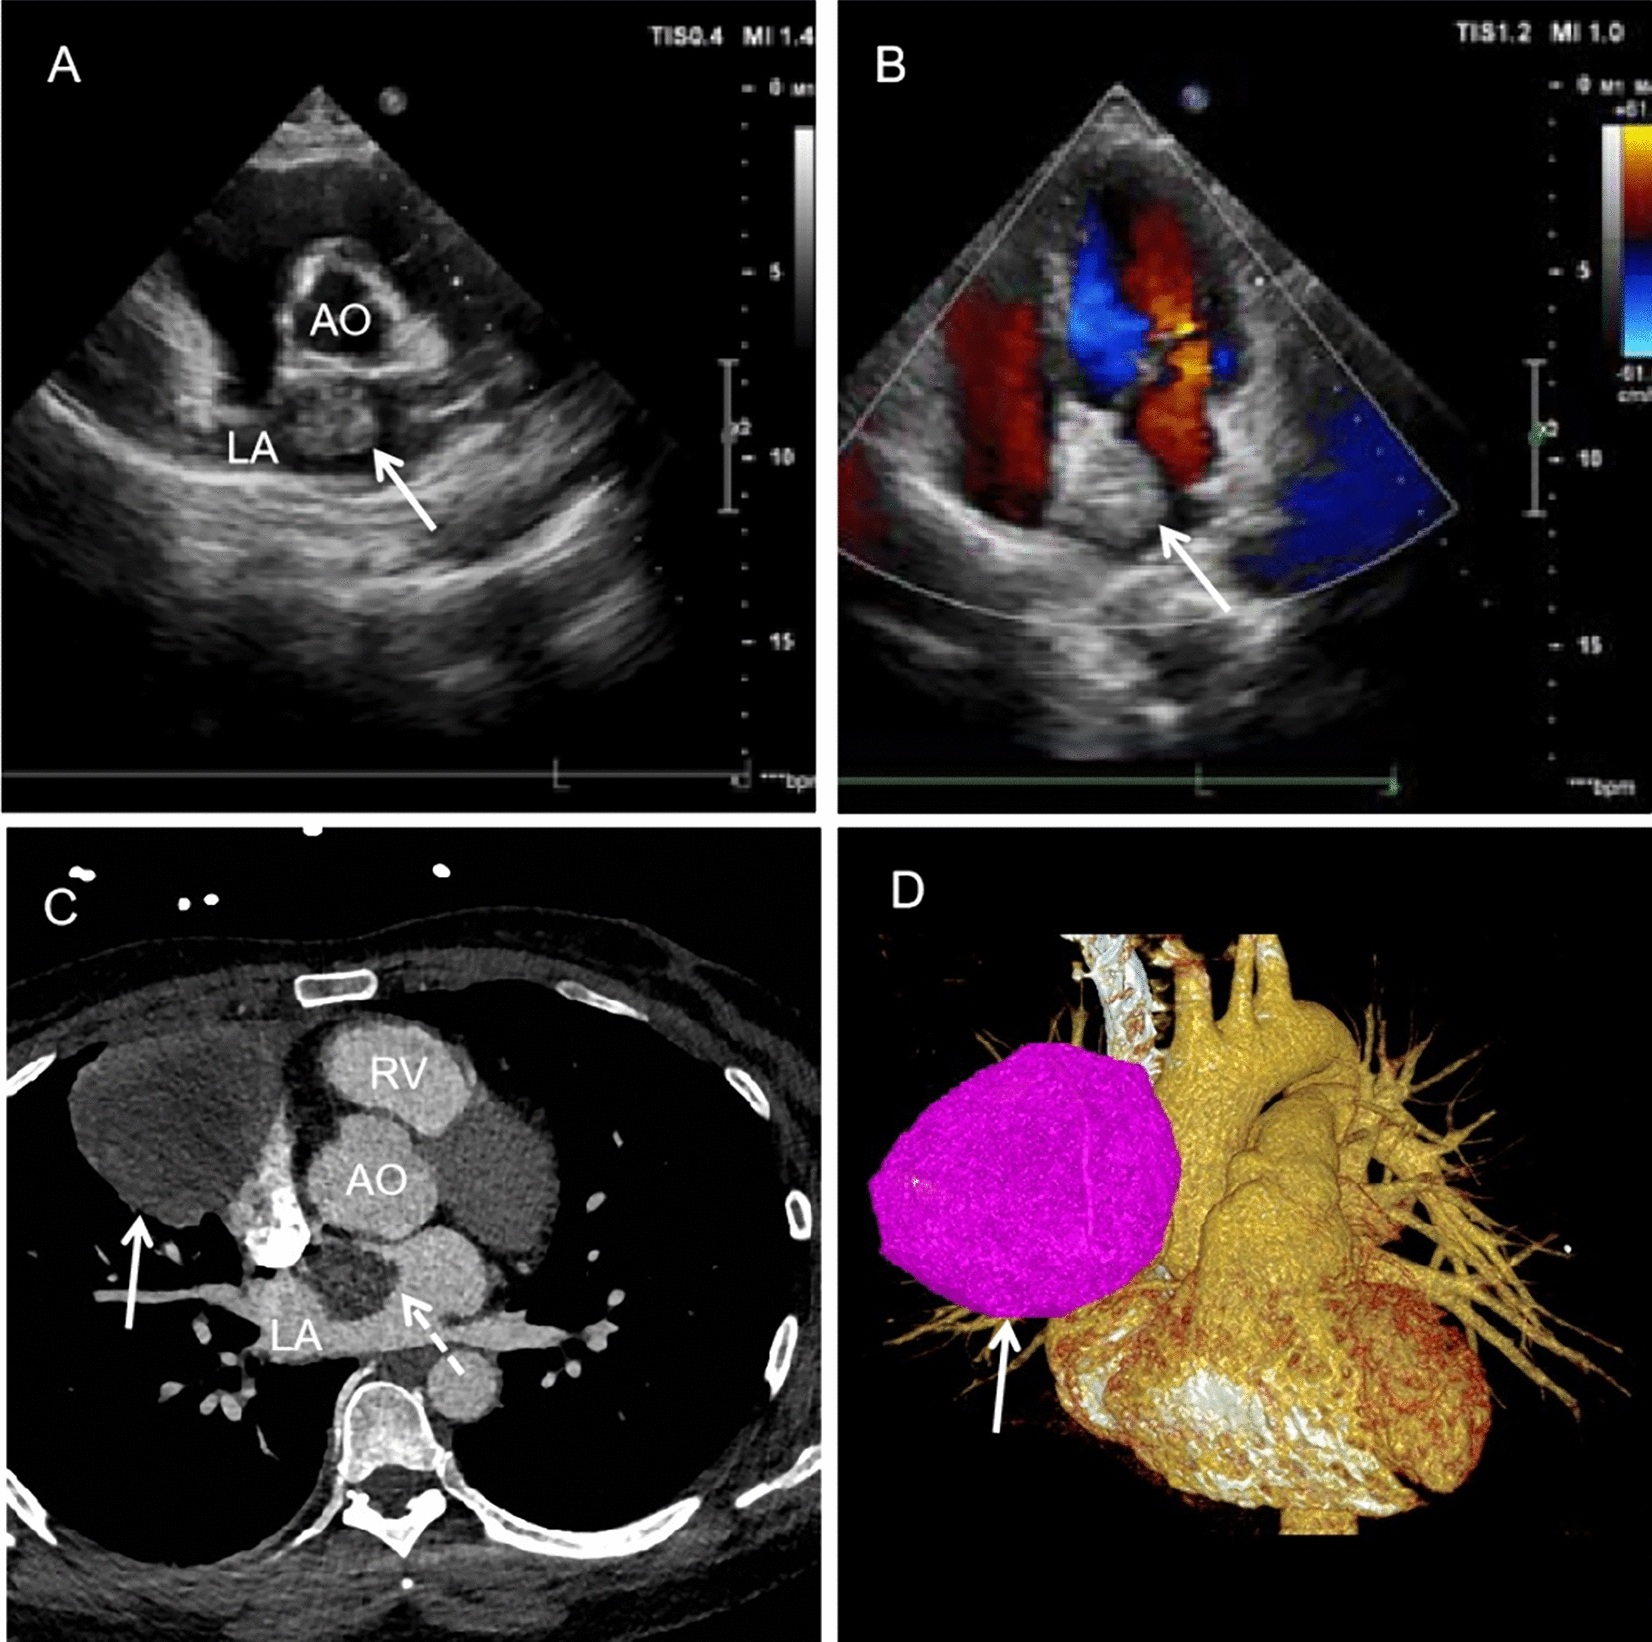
A Cardiac echo and color Doppler blood flow image (B) from left atrium angle, white arrow indicates the tumor. C Contrast-enhanced CT angiography image from transversal axis, white arrow indicates the right anterior mediastinum mass, white dotted arrow indicates the left atrium tumor. D 3D reconstruction image, white arrow indicates the right anterior mediastinum mass. *LA* left atrium, *AO* aortic, *RV* right ventricle
